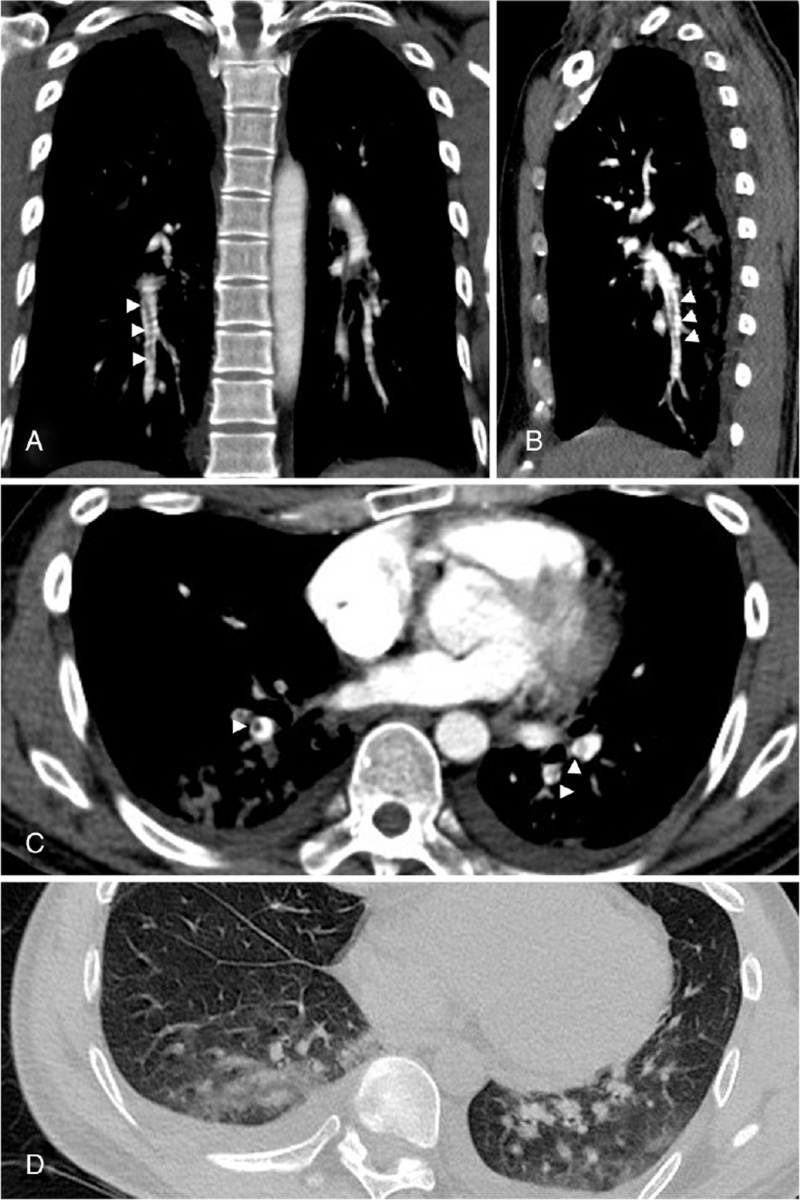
Computed tomography pulmonary angiography showed lower pulmonary artery embolisms (arrowheads) (A) coronal view (B) sagittal view (C) axial view and (D) lung window showed ground-glass opacities in bilateral lung.
